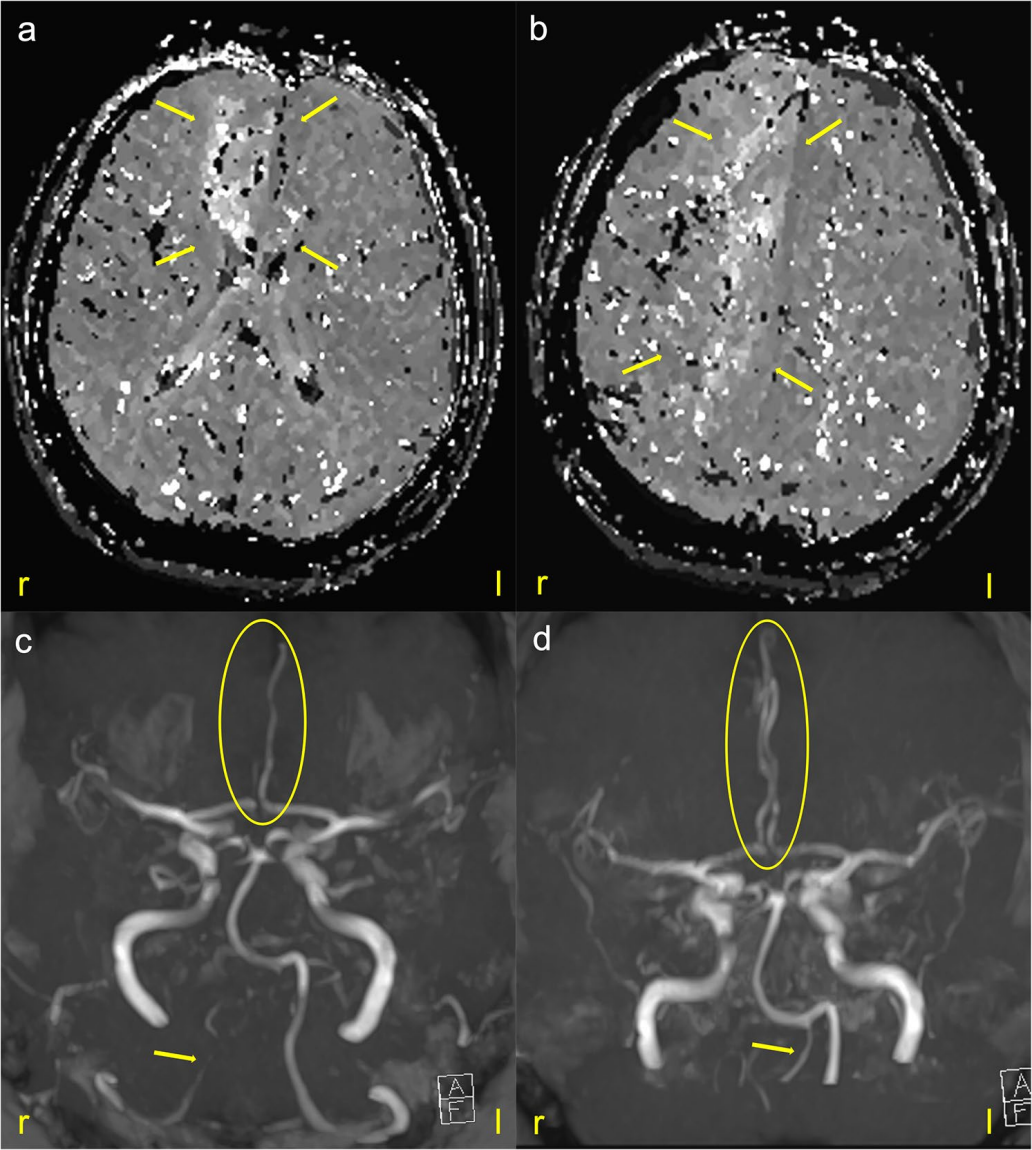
Magnetic resonance imaging (MRI) at a further timepoint. An acute leg-stressed left hemiparesis with aphasia appeared again. Perfusion-weighted imaging (PWI) showed extensive perfusion delay in the right anterior cerebral artery, marked with yellow arrows (a, b). In the MR-time of flight (TOF) angiography, the right anterior cerebral artery can no longer be visualized from the A2-section (c) (yellow ellipse). The right vertebral artery also shows only a weak signal (yellow arrow) resembling a vessel narrowing with an intense left vertebral artery. During intravenous administration of nimodipine, both vessels could be visualized again (d) with a regular flow profile (yellow ellipse and arrow)
